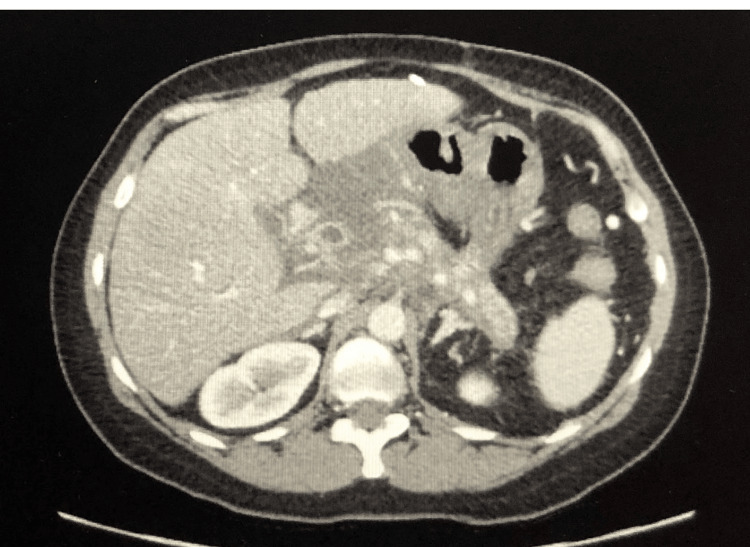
CT of the abdomen confirming the diagnosis of pancreatitis Impression: CT findings compatible with severe acute pancreatitis with extensive pancreatic inflammatory changes and pockets of ill-defined fluid CT: computed tomography
